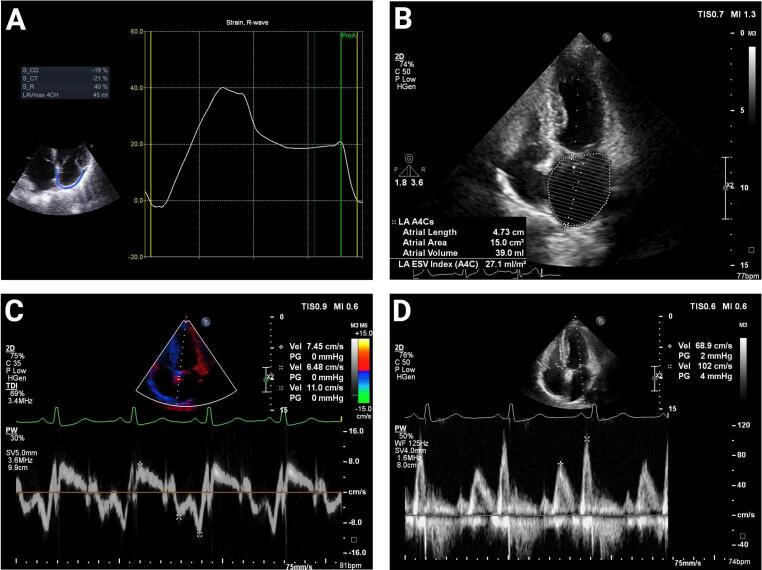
Parameters for the assessment of left ventricular diastolic dysfunction. Panel A: Left atrial strain. Panel B: Assessment of left atrial volume index (LAVI). Panel C: Tissue Doppler imaging–derived early diastolic mitral annular velocity (e′). Panel D: Pulsed-wave Doppler assessment of transmitral inflow velocities
